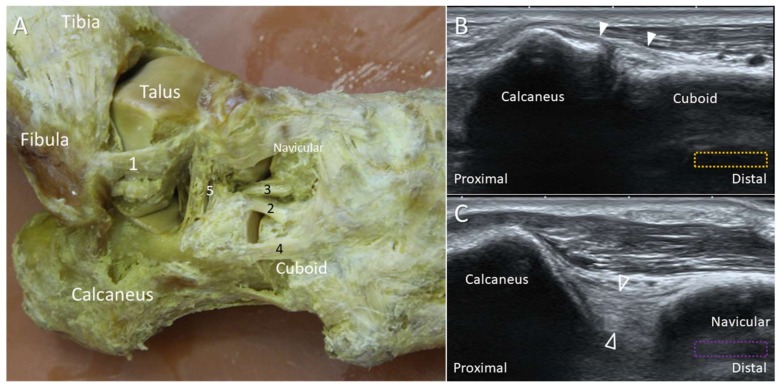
The cadaveric model (A) and ultrasound images (B,C) of the calcaneocuboid (white arrowheads) and calcaneonavicular (void arrowheads) ligaments. The colored dashed rectangles are in accordance with the transducer positions in Figure 2A. 1: Anterior talofibular ligament (ATFL); 2: calcaneocuboid ligament; 3: calcaneonavicular ligament (CNL); 4: lateral calcaneocuboid ligament; 5: cervical ligament.
